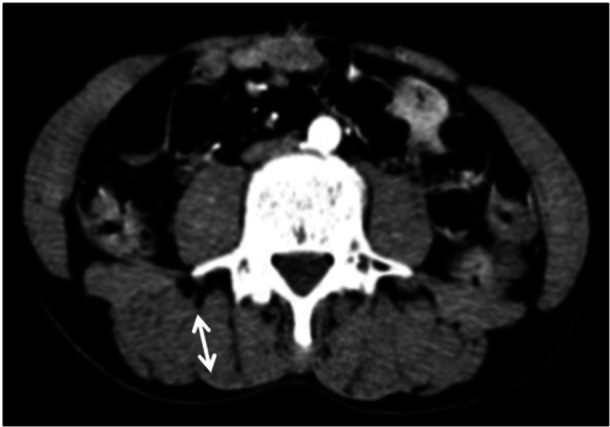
CT imaging in Case 1: Subtle abnormality despite severe endoscopic findings. CT scan of the abdomen shows a soft tissue nodule in the left lower abdomen (white arrow). Differential considerations included inflammatory cellulitis, granuloma, or tumor.
